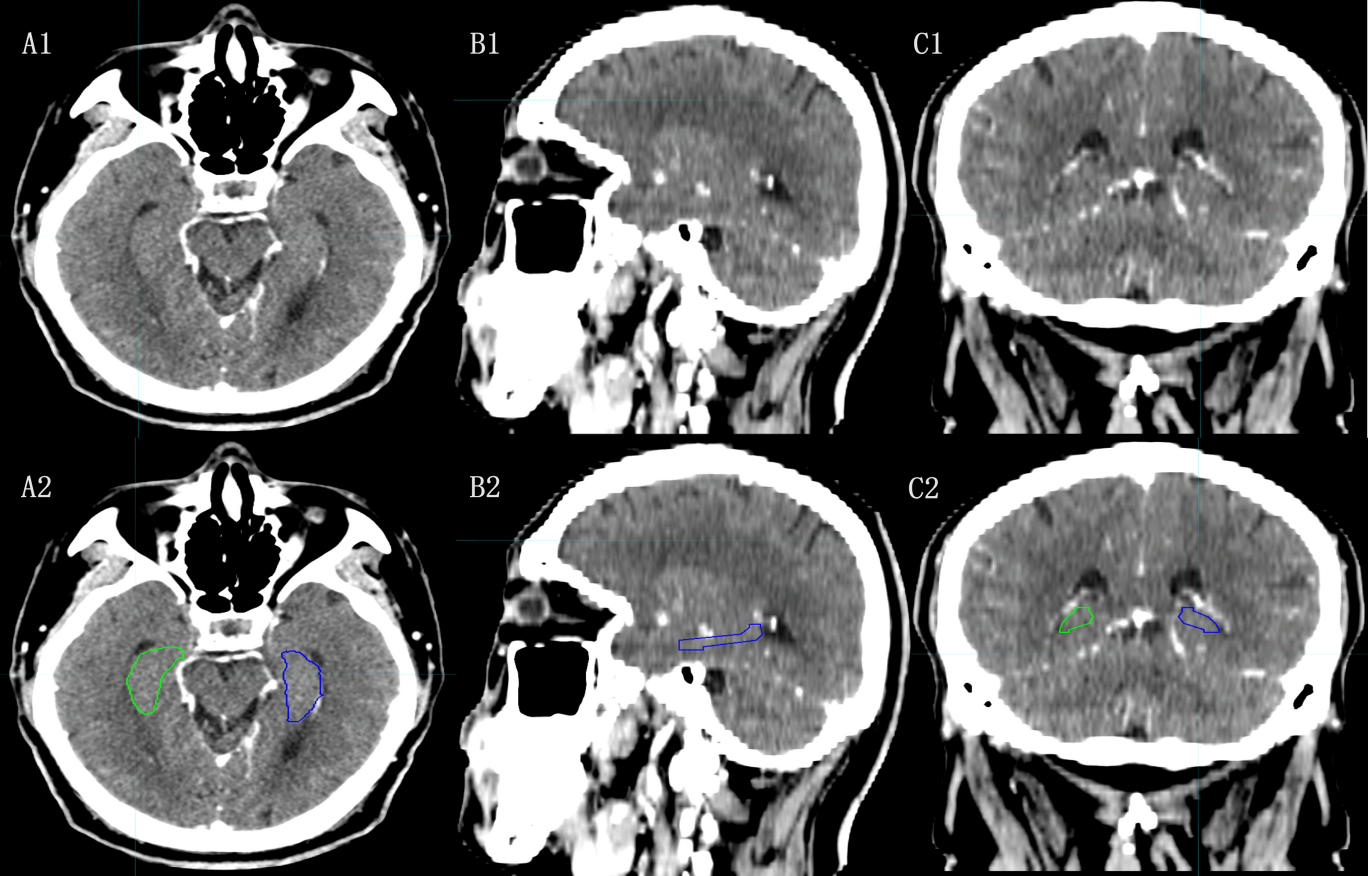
The hippocampal contour based on CT images. [(A1), (B1), (C1):Axial, Sagital and Coronal images of CT; (A2), (B2), (C2): Axial, Sagital, Coronal images of CT with hippocampal contour].
